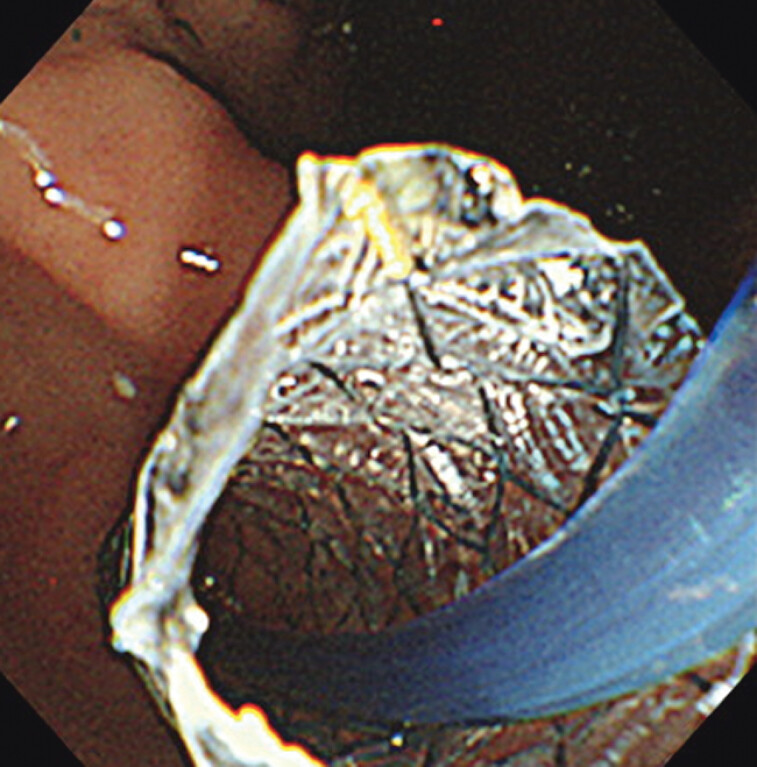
Insertion of a self-expandable metal stent via the endosonographically created route.
